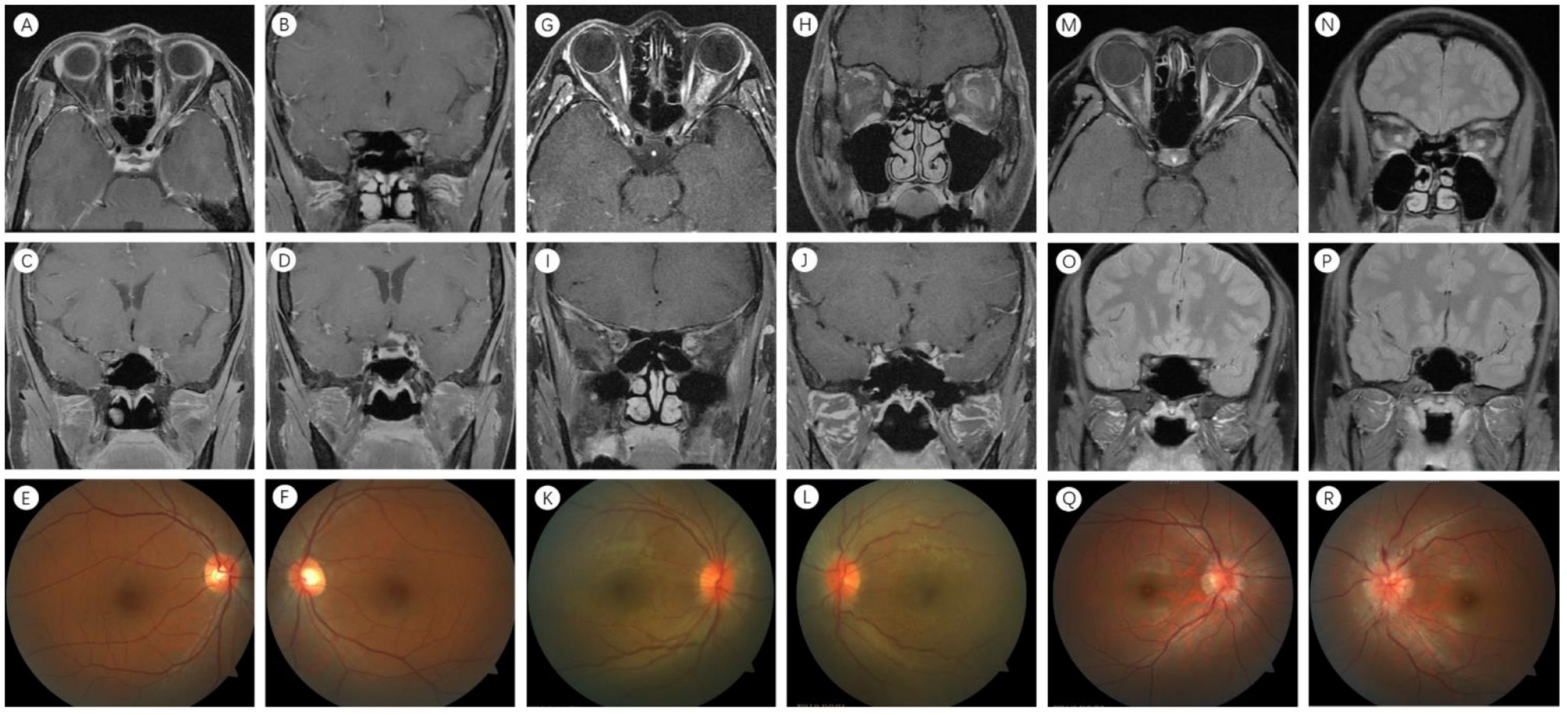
Neuroimaging features in NMO-ON, MOG-ON, and COVID-19 ON. (A–F) Left eye in NMO-ON. (A) Orbital MRI: orbital enhancement. (B) Orbital MRI: canalicular enhancement. (C) Orbital MRI: intracranial enhancement. (D) Orbital MRI: optic chiasm enhancement. (E, F) No abnormalities in bilateral optic nerve intraocular segments. (G–L) Left eye in MOG-ON. (G) Orbital MRI: orbital enhancement. (H, I) Orbital MRI: orbital enhancement with meningeal enhancement. (J) Orbital MRI: intracranial enhancement. (K) No abnormalities in the right optic nerve intraocular segment. (L) Left optic nerve intraocular segment swelling. (M–R) Both eyes in COVID-19-ON. (M, N) Orbital MRI: bilateral orbital enhancement. (O) Orbital MRI: bilateral canalicular enhancement. (P) Orbital MRI: no abnormal intracranial enhancement. (Q, R) Bilateral optic nerve intraocular segment swelling. NMO-ON, neuromyelitis optica-related optic neuritis; MOG-ON, myelin oligodendrocyte glycoprotein optic neuritis; COVID-19 ON, optic neuritis associated with COVID-19; MRI, magnetic resonance imaging.
